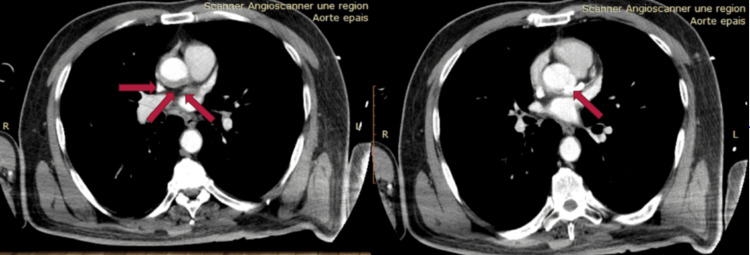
Aortic computed tomography angiography The red arrow shows circumferential transmural hematoma at the sino-tubular junction to be monitored. The stent is in place and there is no aortic dissection.
